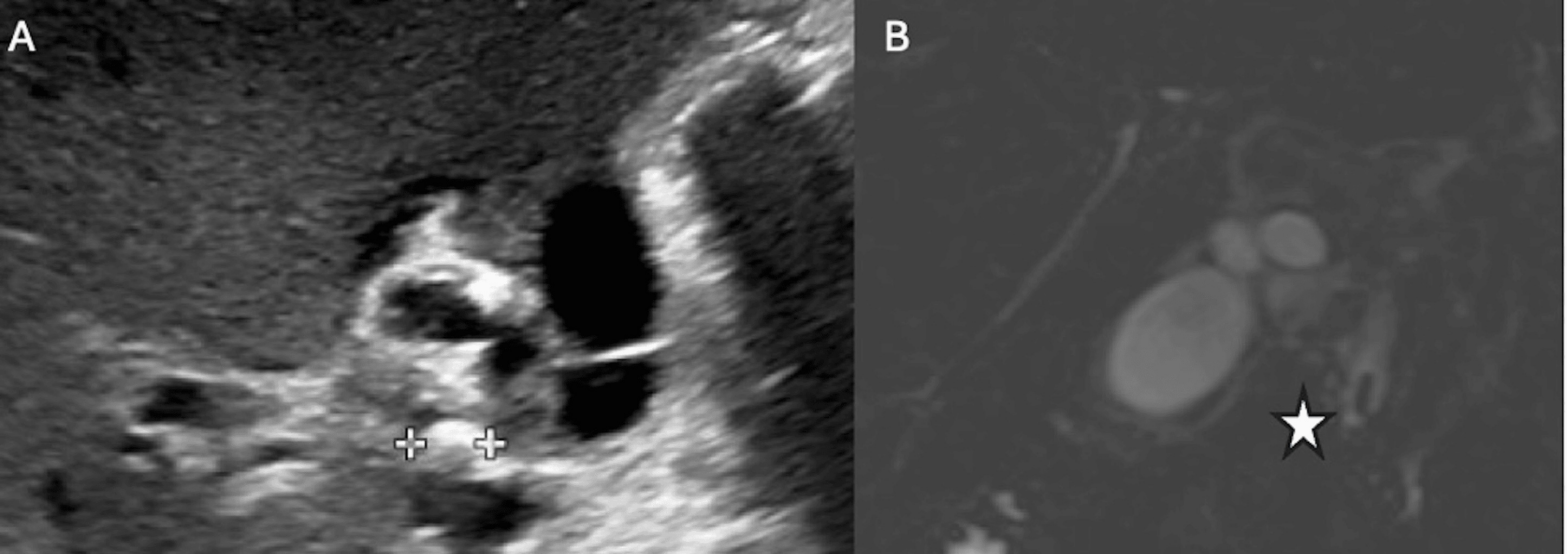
Biliary imaging for case presentation one A) Abdominal ultrasound showing a gallstone within the cystic duct. Crosshairs indicate the width of the gallstone. B) Magnetic resonance cholangiopancreatography showing a 6 mm stone within the common bile duct. The star demonstrates the location of the stone.
